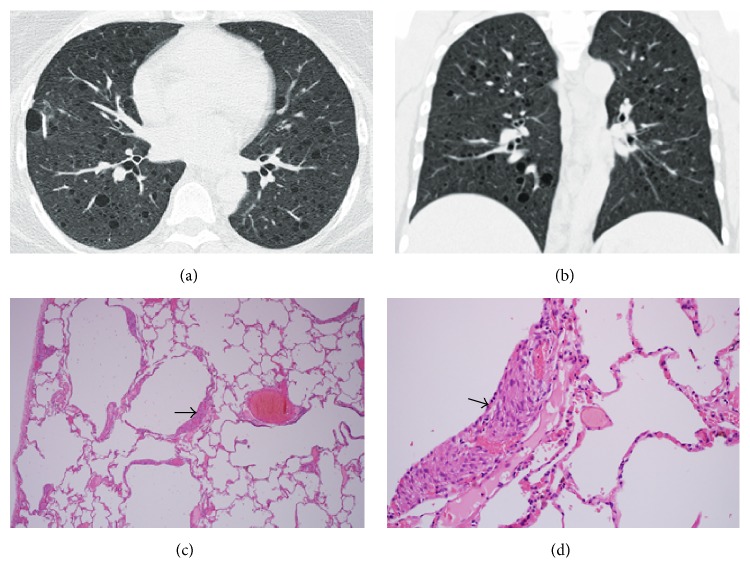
Lymphangioleiomyomatosis (LAM). High-resolution CT scan in axial (a) and coronal (b) views demonstrating numerous well-defined, thin-walled lung cysts varying in size from 2 to 18 mm. There is diffuse involvement of all lung fields, and the lung parenchyma between the cysts is normal. (c) Thin-walled cystic air spaces interspersed between uninvolved lung parenchymata (magnification ×40). (d) Focal cyst wall thickening from smooth muscle proliferation (arrow) (magnification ×100).
